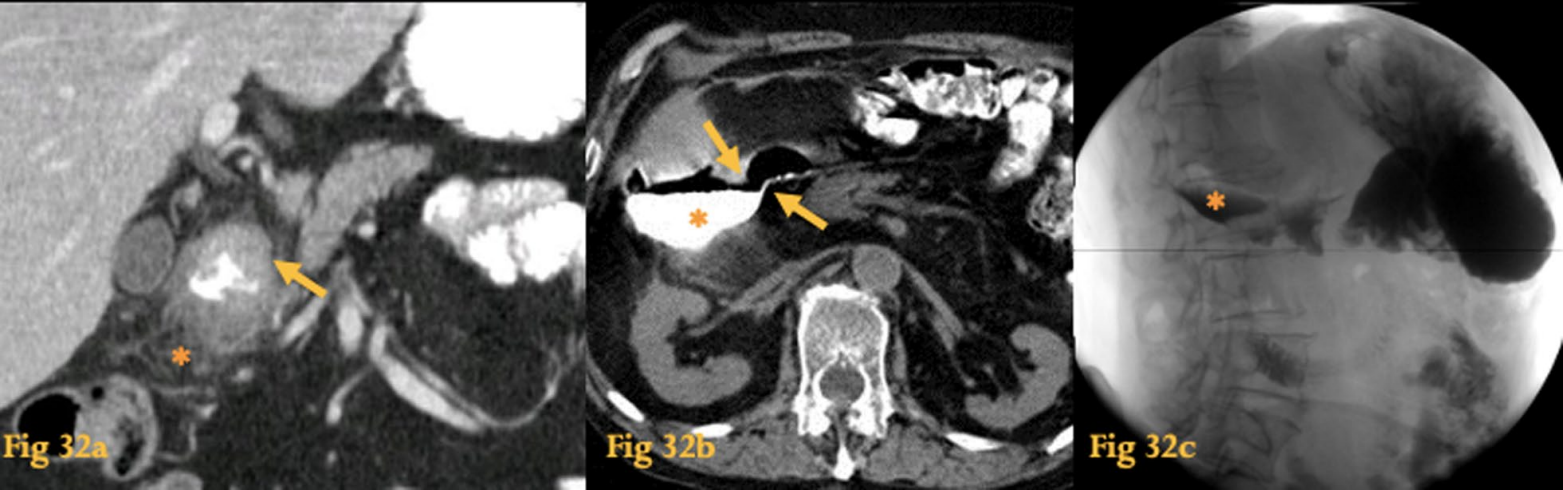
Ulcers and duodenitis. Abdominal CT of a case of duodenitis (a): the lumen of the duodenum is irregular; the wall is thickened (arrow) and there is adjacent fat stranding (asterisk). Plain abdominal CT of another patient (b) clearly shows a duodenal ulcer perforation (arrows) and accumulation of gas and oral contrast in the right anterior pararenal space (asterisk). The corresponding upper GI study performed with water-soluble contrast agent (c) shows retroperitoneal leakage of contrast medium (asterisk)
